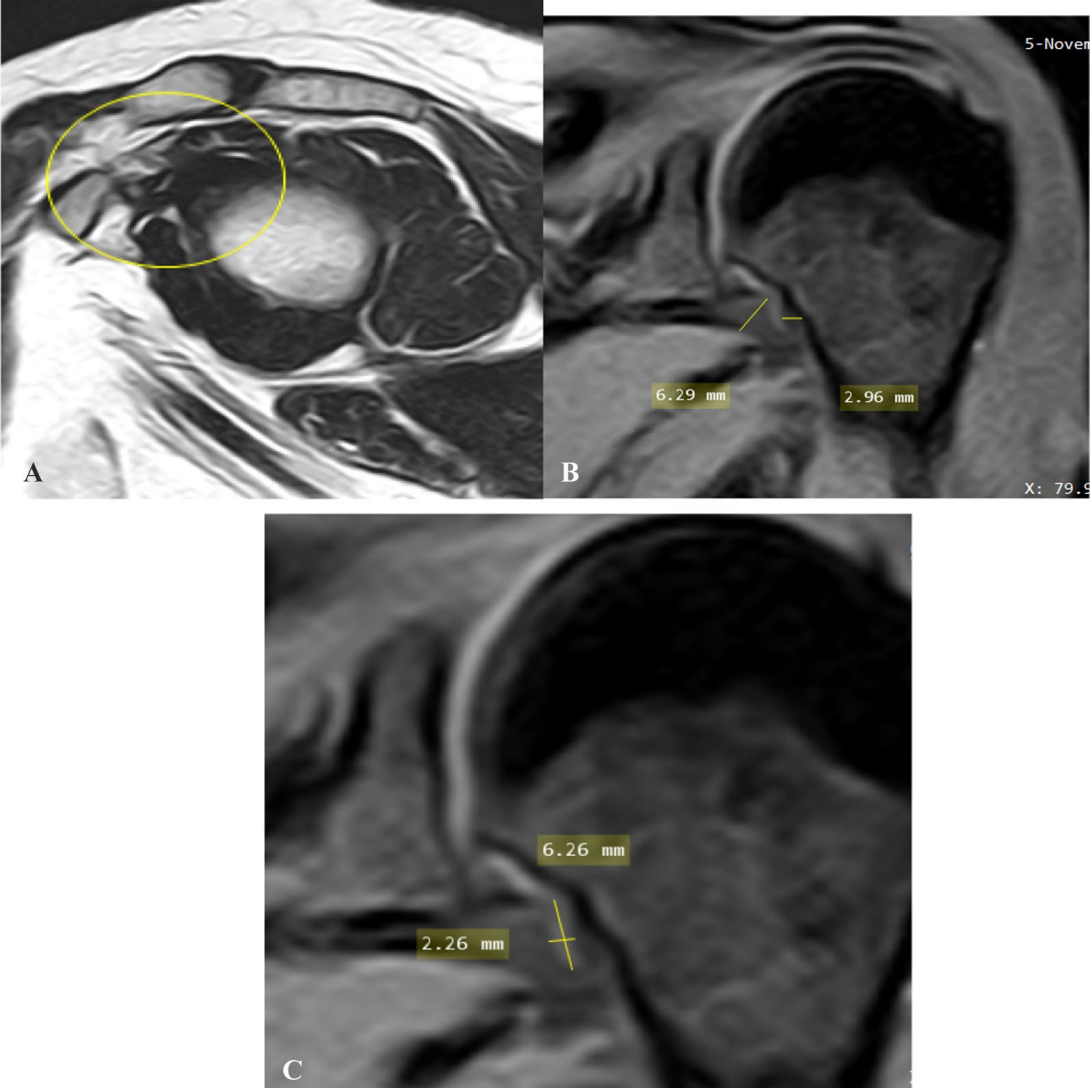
A Oblique sagittal T2-weighted MR image shows partial obliteration of subcoracoid fat triangle with no observed fat planes or coracohumeral ligament (encircled area). B Oblique coronal fat-suppressed PD-weighted MR image shows maximum capsule thickness at glenoid and humeral portions of axillary recess, the humeral was fibrotic and obliterated. C Oblique coronal fat-suppressed PD-weighted MR image shows measurement of maximal height and width of axillary recess
